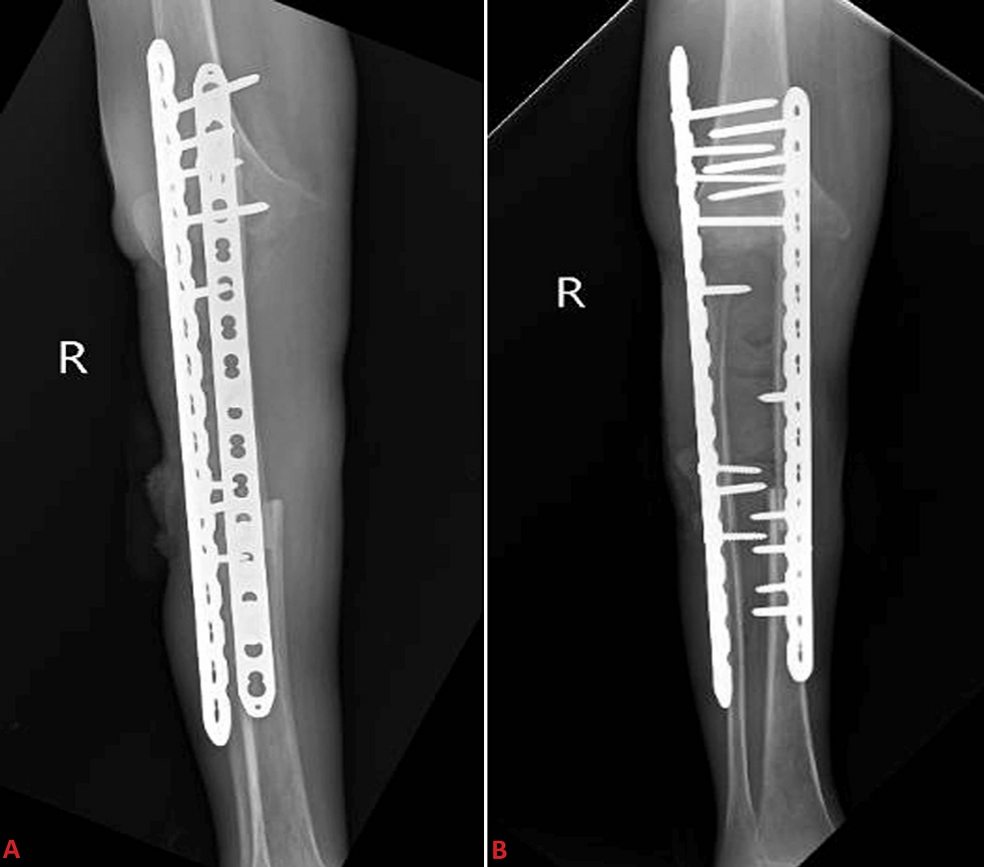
Postop X-ray of the right knee with leg
